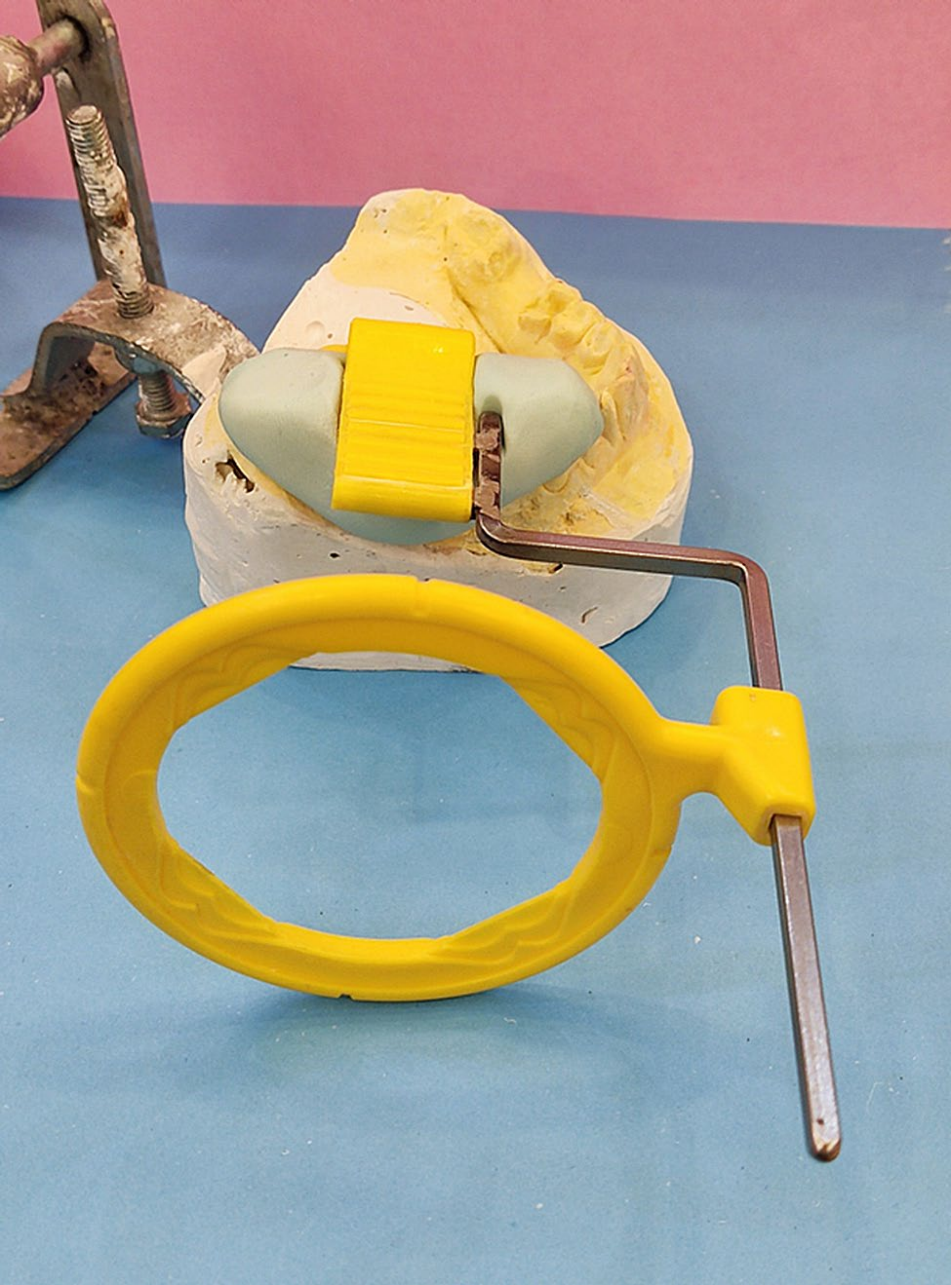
Film holder and putty index for the repeatable position for taking radiographs
